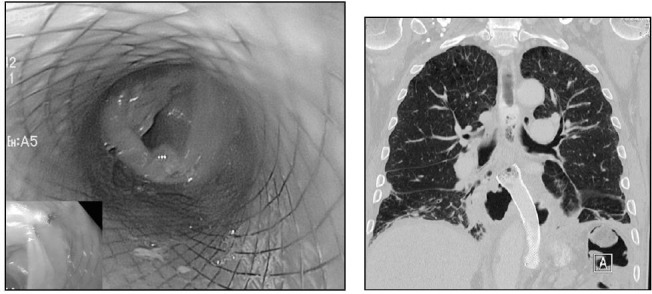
Endoscopic stent insertion for leakage of an oesophagojejunostomy: The endoscopic image (left) shows the already inserted stent and the intestinal mucosa of the jejunum is visible at the caudal end of the stent. The computed tomography (right) shows the stent in the inferior mediastinum. The stent’s position is much more distal than in Figure 1 because the oesophagojejunostomy is routinely performed inferior of the tracheal bifurcation.
